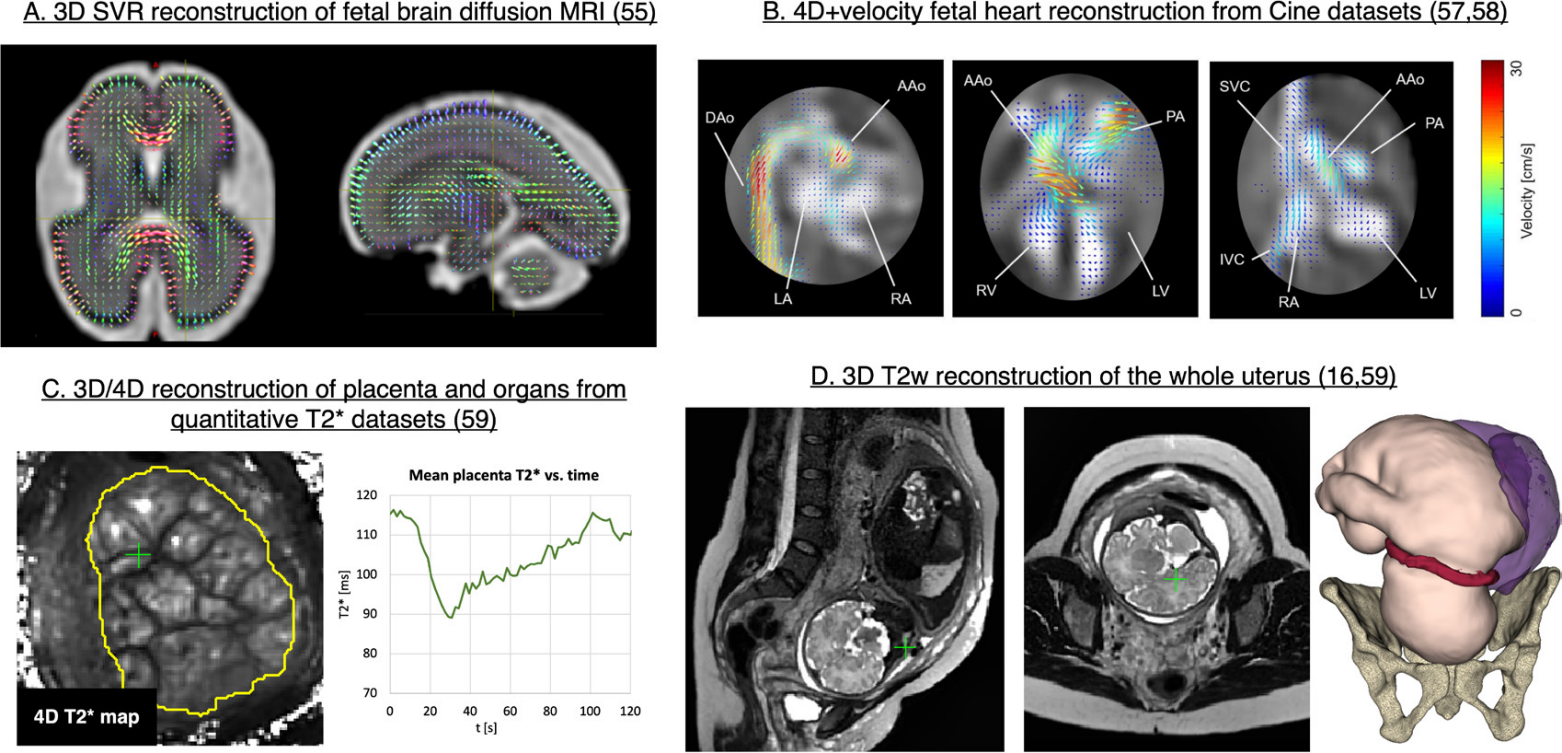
Examples of SVR-based solutions for advanced foetal MRI acquisition methods. SVR, slice-to-volume registration.
